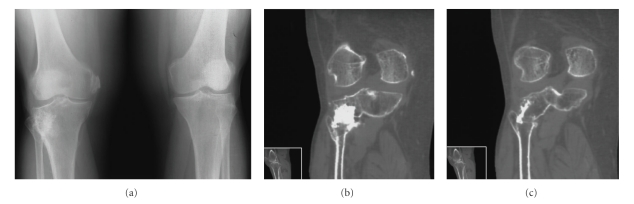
AP radiograph of both knees (a), and CT (b,c) examination in a 69-year-old woman, revealing ectopic calcification of the proximal TFJ of the right knee.
